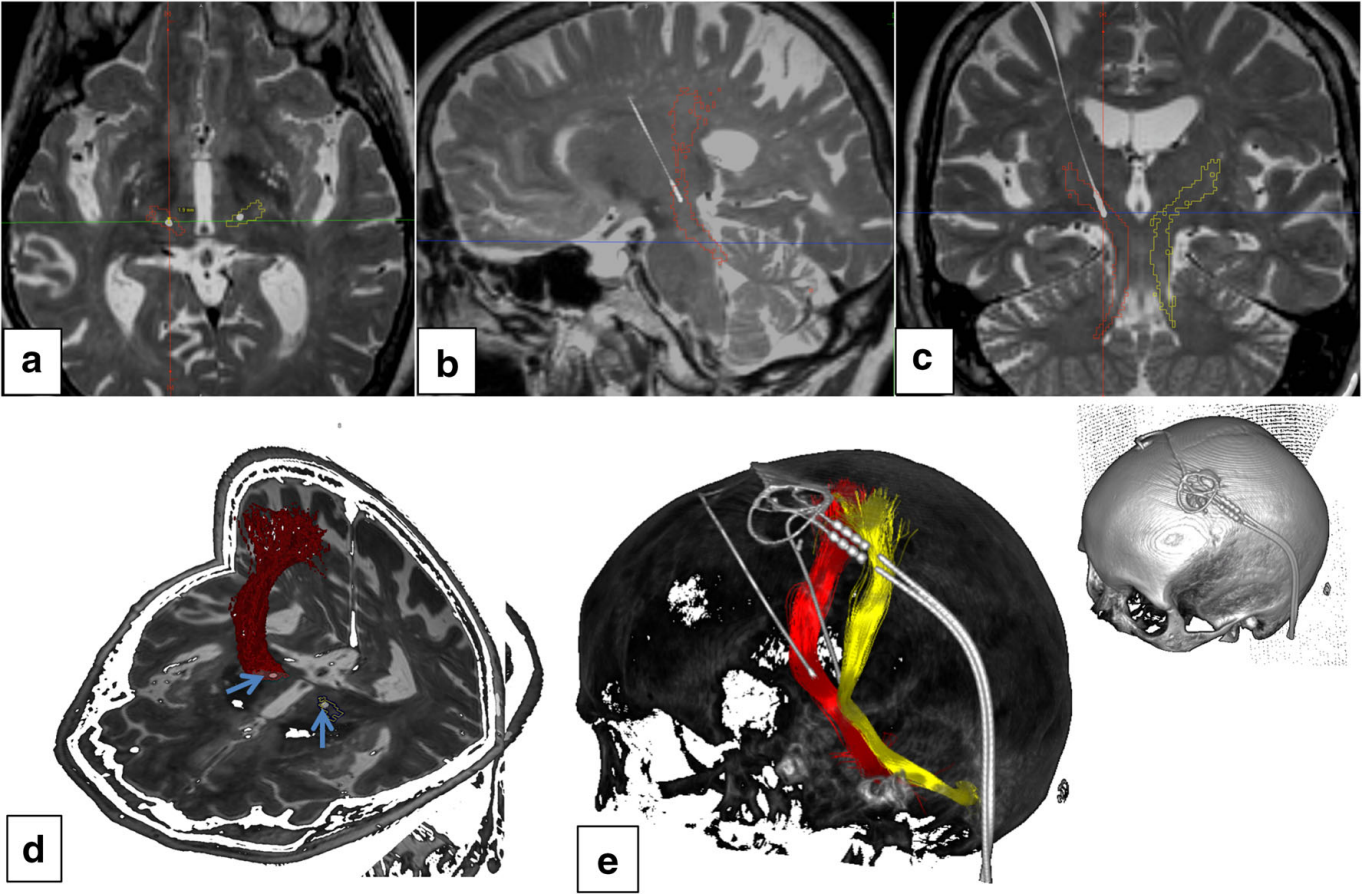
Clinical example of typical Vim/DRT DBS. a–c Axial, sagittal, and coronal depiction, respectively. T2-weighted MRI, electrodes superimposed from helical CT. d Quasi three-dimensional view from anterior superior and left. Blue arrows indicate DBS electrodes. e Three-dimensional representation of the tractography result (DRT: left = yellow; right = red)
